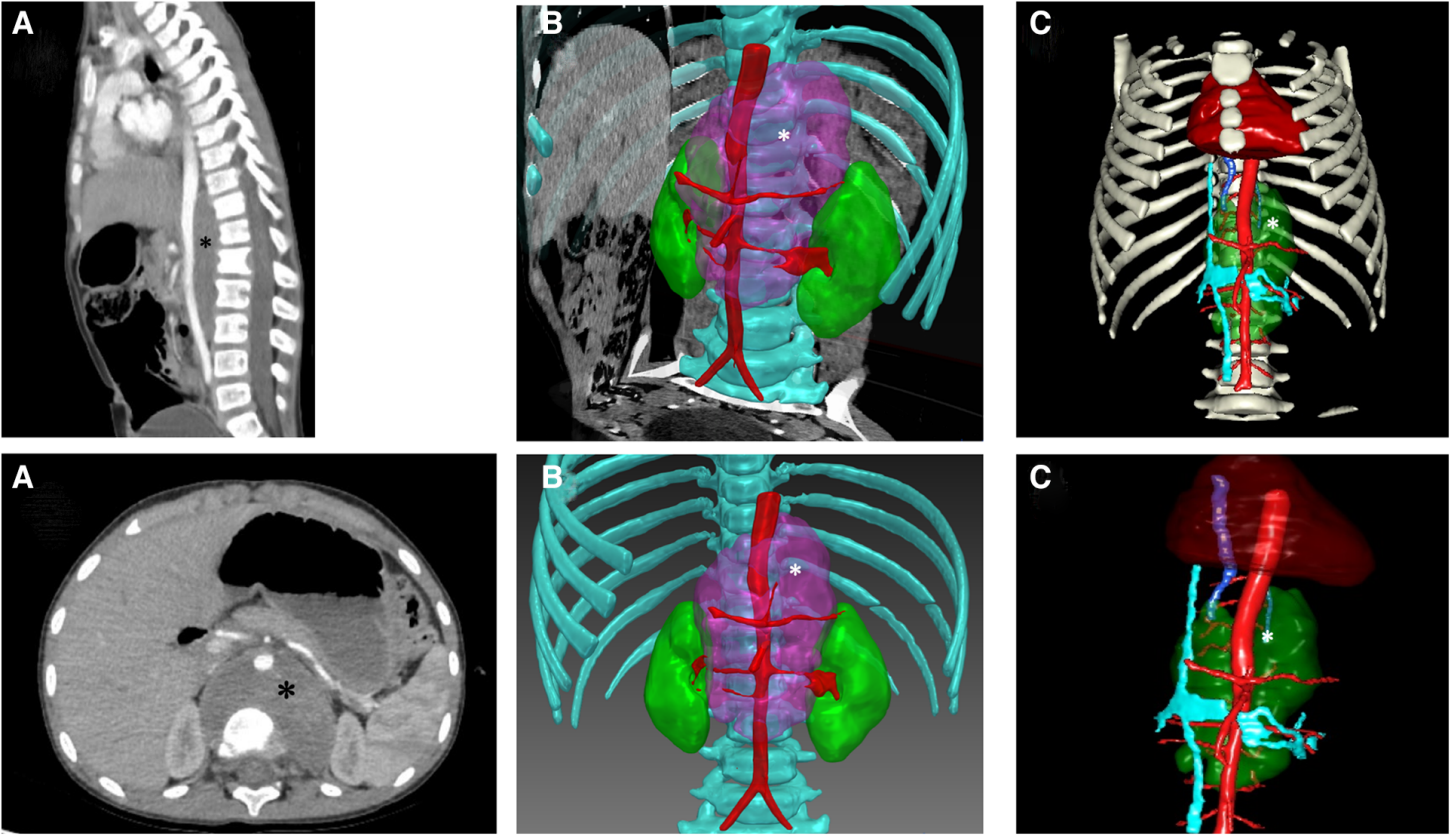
Abdominal neuroblastoma and 3D modelling. (A) Computed tomography acquisition of an abdominal median neuroblastoma (*) in a 4-year-old boy. (B) Segmentation of the tumor (*) using Medical Imaging Interaction Toolkit (MITK°-free open source software-German Cancer Research-Germany) located between vertebra and aorta. (C) Segmentation of the neuroblastoma (*) and surrounding structures by Visible Patient° software (Visible Patient°-Strasbourg-France).
